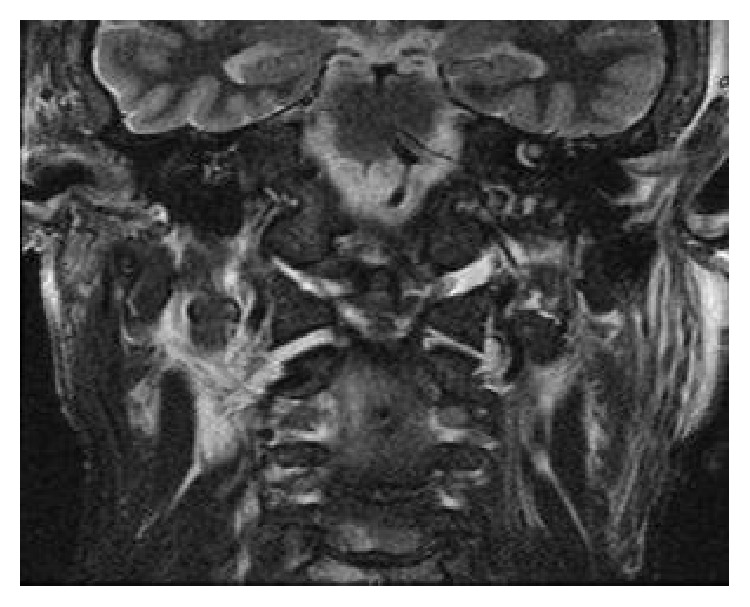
Coronal T2 weighted MRI showing occiput-C1 displacement, C1-C2 displacement, and soft tissue edema in the pericervical spine region.
